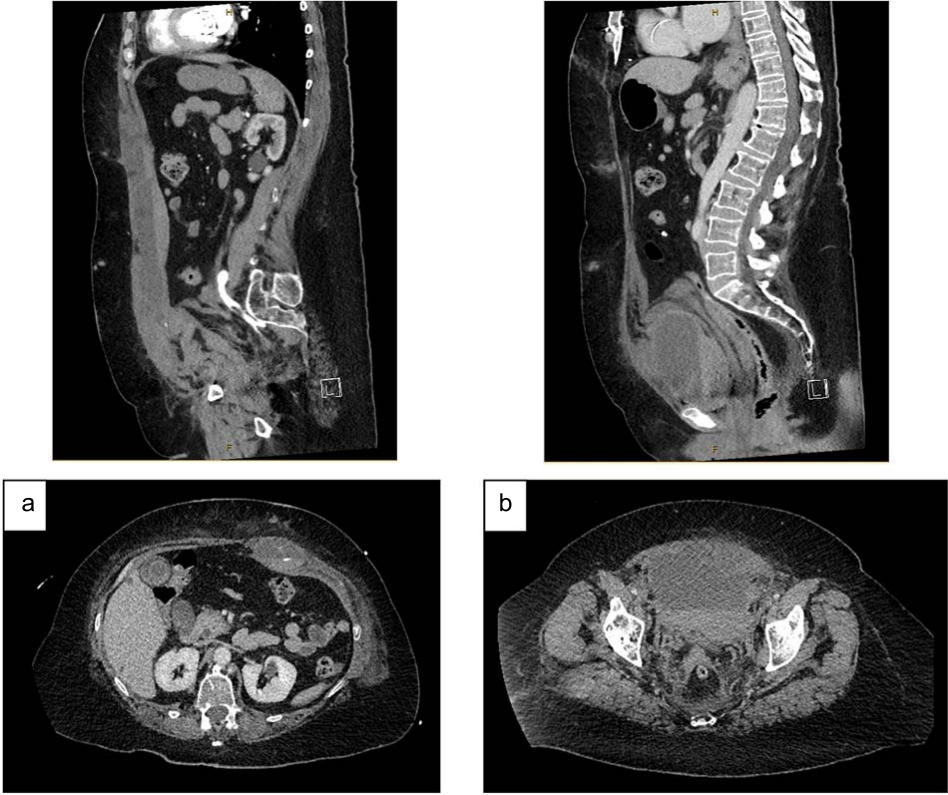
CT angiography (portal venous phase): (a) increased size of RSH and ongoing active extravasation – sagittal (top) and axial (bottom) views. (b) Extraperitoneal haematoma compressing and displacing urinary bladder – sagittal (top) and axial (bottom) views.
